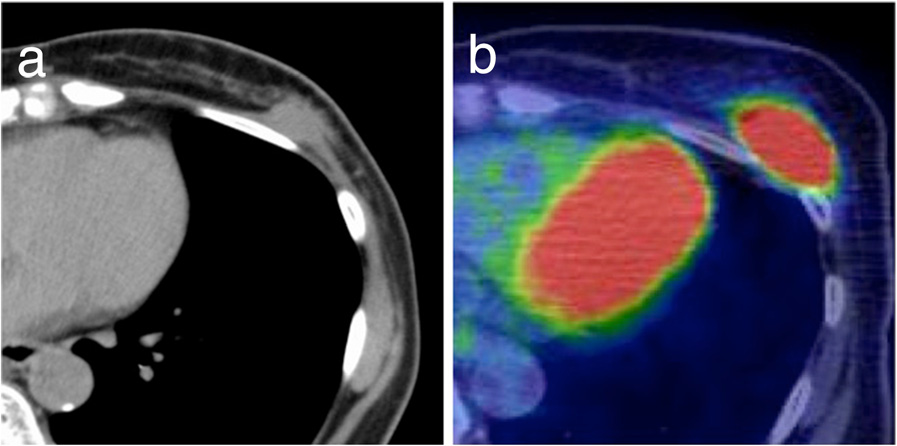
Imaging findings. On positron emission tomography (PET), the maximum standardized uptake value was 12.7, which corresponded to the tumor finding on computed tomography (CT). a CT. b PET
